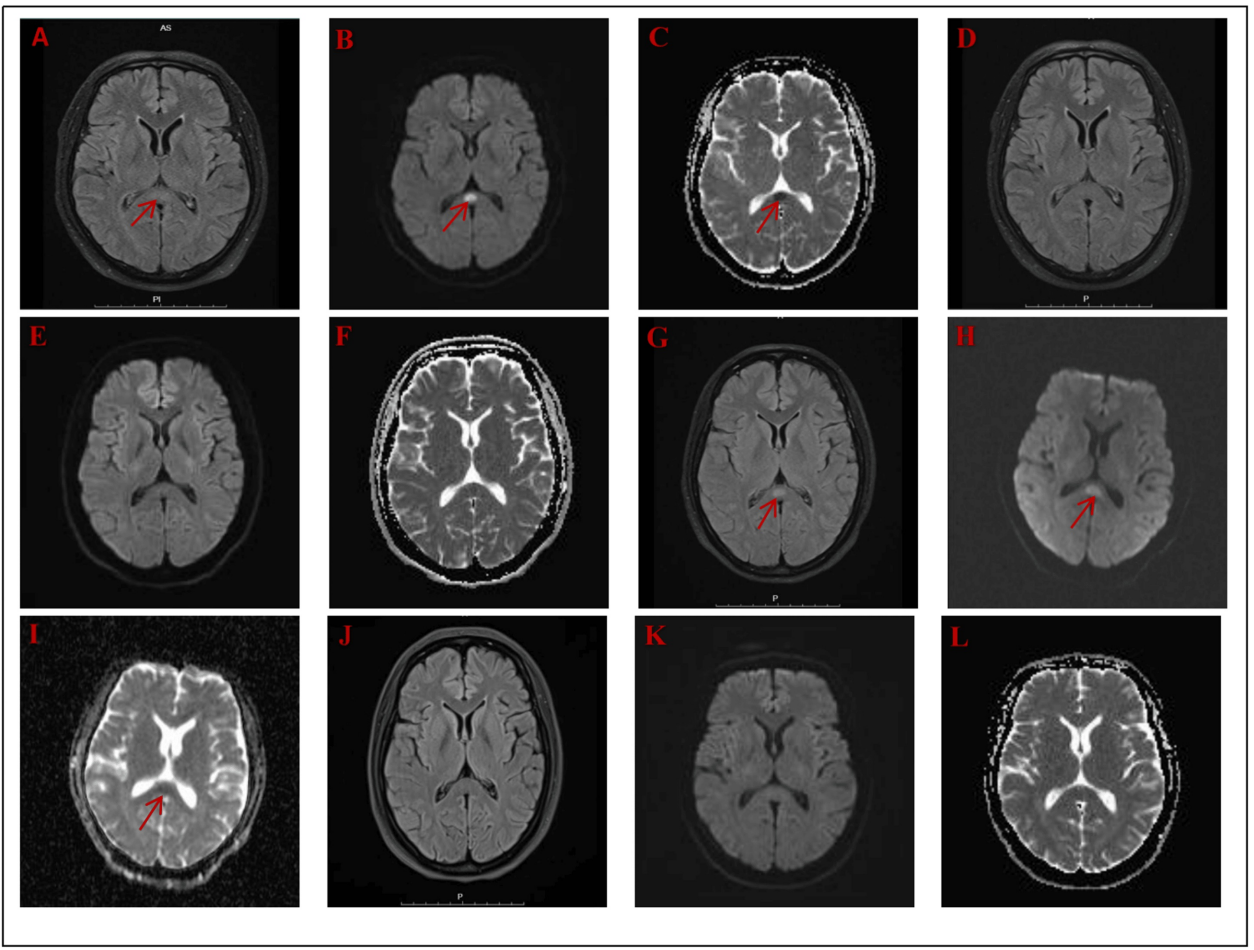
Cerebral MRI of two cases. On the day of admission, the MRI of Case 1 showed a slightly hyperintense signal on FLAIR (A), a hyperintense signal on DWI (B), and a hypointense signal on ADC (C) in the SCC. After treatment, FLAIR (D), DWI (E), and ADC (F) showed that the splenial lesion had almost disappeared in the same area. On the day of admission, the FLAIR (G) and DWI sequences (H) of Case 2 showed hyperintense lesions in the corpus callosum, and the ADC values within the lesions were low (I). After treatment, FLAIR (J), DWI (K), and ADC (L) showed that the splenial lesion on the follow-up MRI had disappeared. FLAIR: fluid-attenuated inversion recovery; DWI: diffusion-weighted imaging; ADC: apparent diffusion coefficient; MRI: magnetic resonance imaging; SCC: splenium of the corpus callosum
